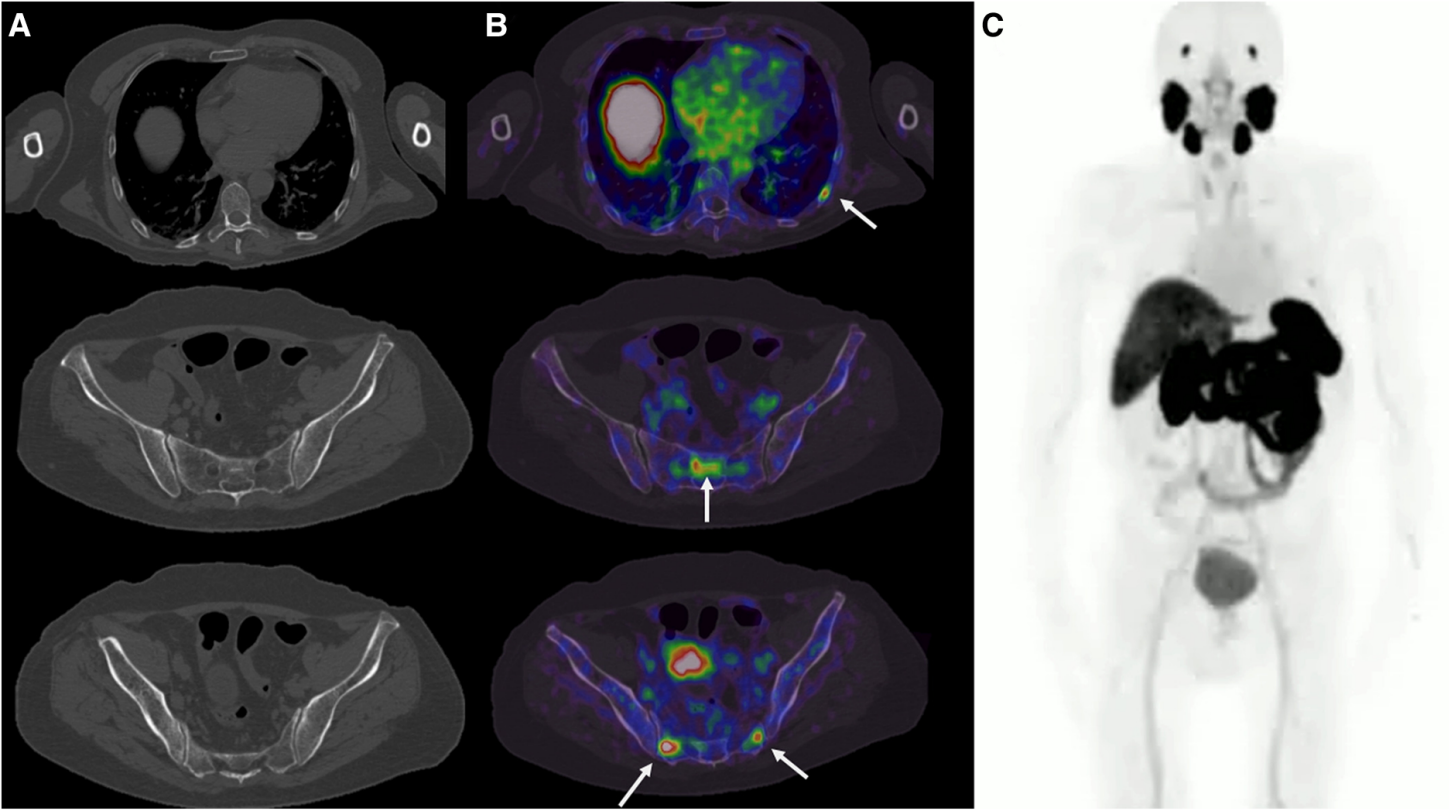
18F-PSMA-1007 PET/CT. (A) Axial slices of plain tomography without blastic bone lesions. (B) PET/CT shows focal areas of radiotracer uptake in the left posterior seventh costal arch and sacrum, without morphologic translation. (C) Maximum intensity projection with 18F-PSMA-1007.
